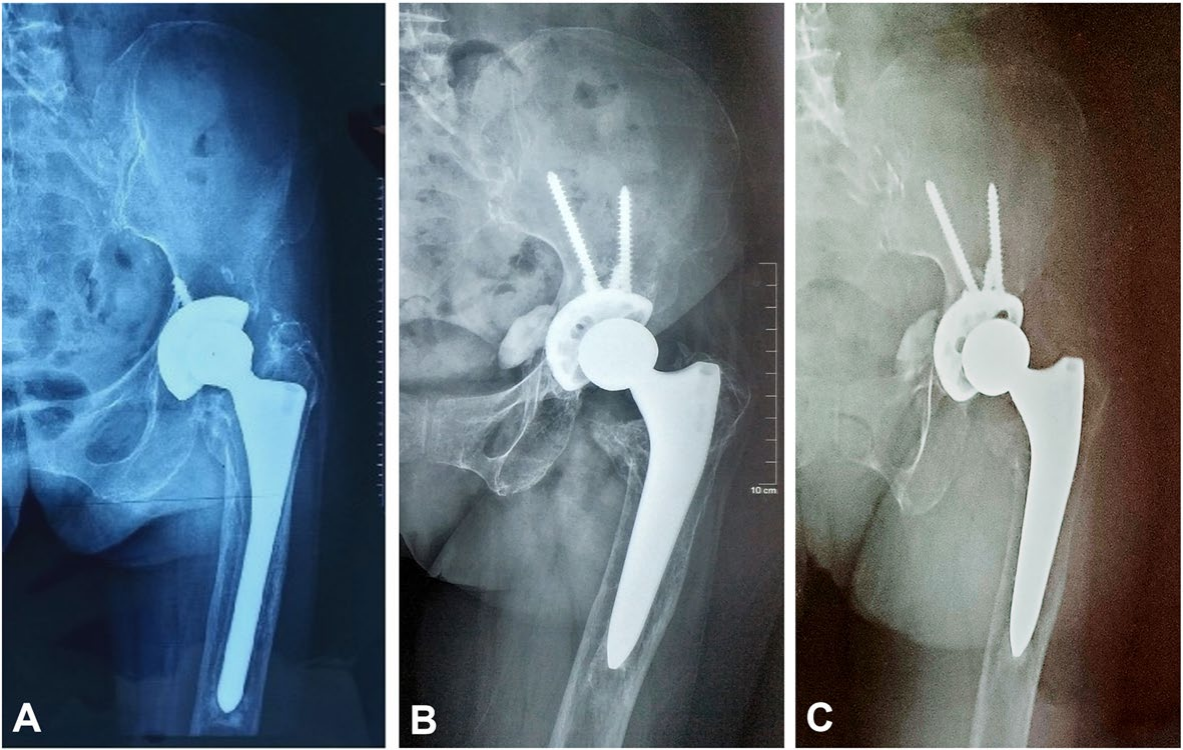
Radiographs show A proximal femoral bone defect (Paprosky IV) in a 68-year-old female patient with aseptic loosening, B, C the fixed cementless SL-Plus MIA stem without osteolysis, subsidence, or loosening at 15- and 71-month follow-up
